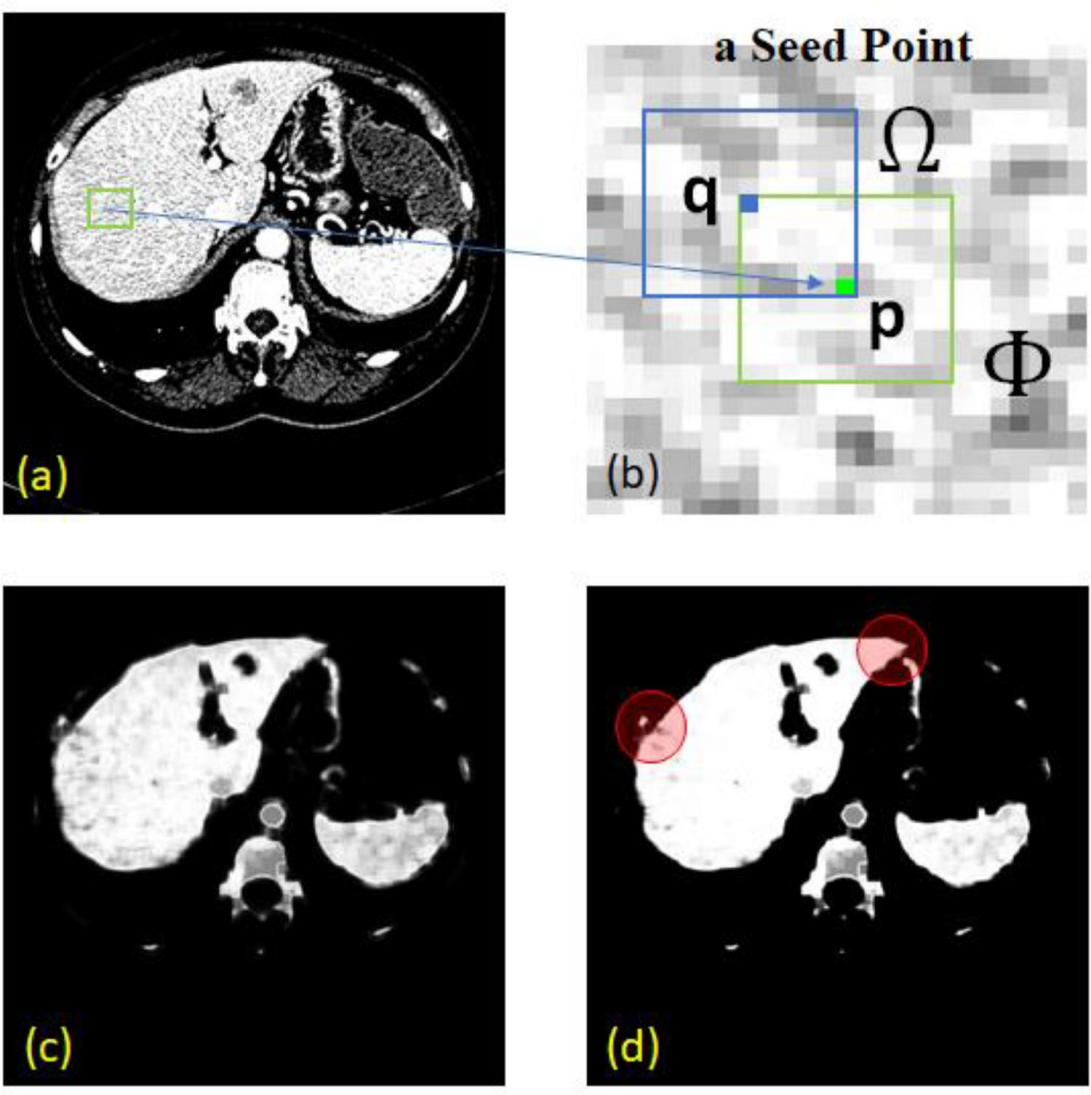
An example of applying probability map and relaxation labeling. The original CT image (a) with a seed point (green) is shown in (b), the probability map image and RL enhanced results are shown in (c) and (d), respectively. The probability values from 0 to 1 are scaled to 0 to 255 for visualization purpose.
